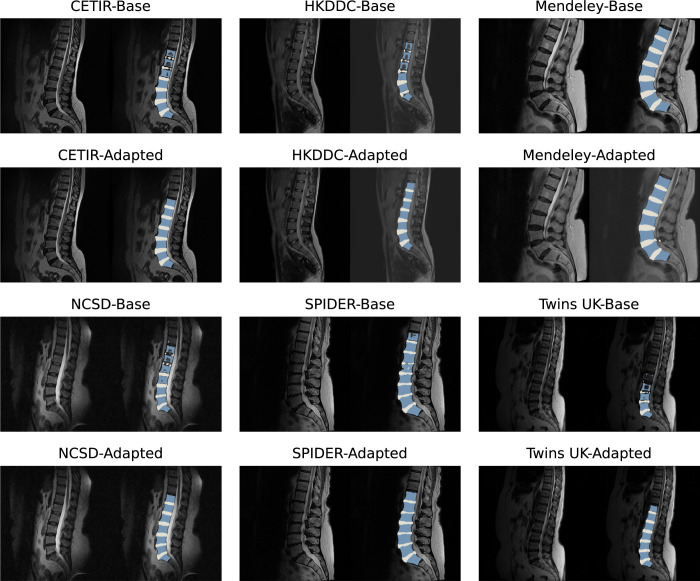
Comparison of segmentation performance between the base and adapted models across 6 external lumbar spine magnetic resonance imaging data sets. The adapted model demonstrates improved generalization providing more precise detection of vertebral bodies and intervertebral discs.
